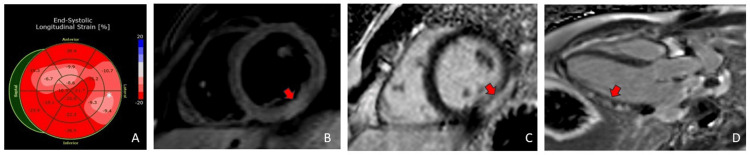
Panel A: Abnormal global longitudinal strain at the lateral wall. Panel B: Hypertense signal on T2-weighted imaging indicating the presence of myocardial edema (red arrow). Panel C: Increased native T1 and extracellular volume (ECV) indicating myocardial necrosis causing extracellular fraction expansion (red arrow). Panel D: Late gadolinium enhancement due to the delayed washout in the diseased myocardium; the injury pattern is most consistent with nonischemic based predominantly on the meso- and epicardial enhancement with relative sparing of the subendocardium (red arrow).
